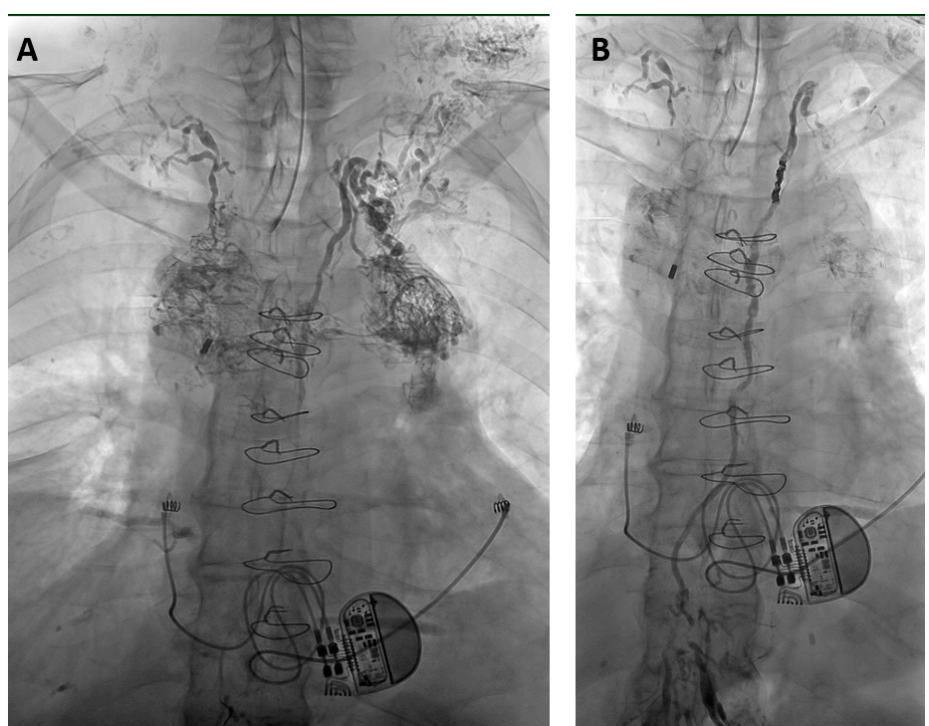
65-year-old man with a non-traumatic bilateral chylothorax and chylopericardium. (A) X-ray lymphangiography shows chylous reflux from the upper thoracic part of the thoracic duct into dilated and tortuous lymphatic vessels in the mediastinum and cervical soft tissue. The right-sided lymphatic duct at the right venous angle can also be seen. (B) After thoracic duct embolization with micro-coils and a mixture of NBCA/iodized oil (ratio 1:3) leakage ceased within 2 days without clinical sequelae over a follow-up time of 4 years.
